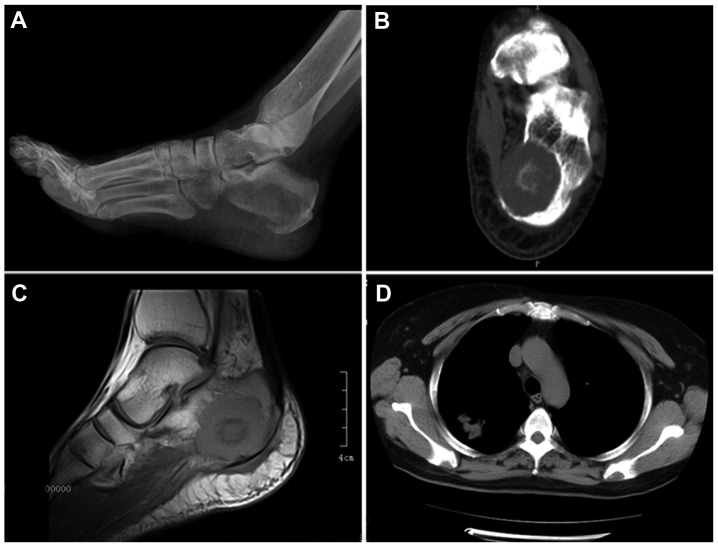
Medical imaging examinations including (A) an X-ray of the left calcaneus; (B) a computed tomography (CT) scan of left calcaneus; (C) a sagittal MR T1-weighted image of the left calcaneus; and (D) chest CT scans of the patient. MR, magnetic resonance.
